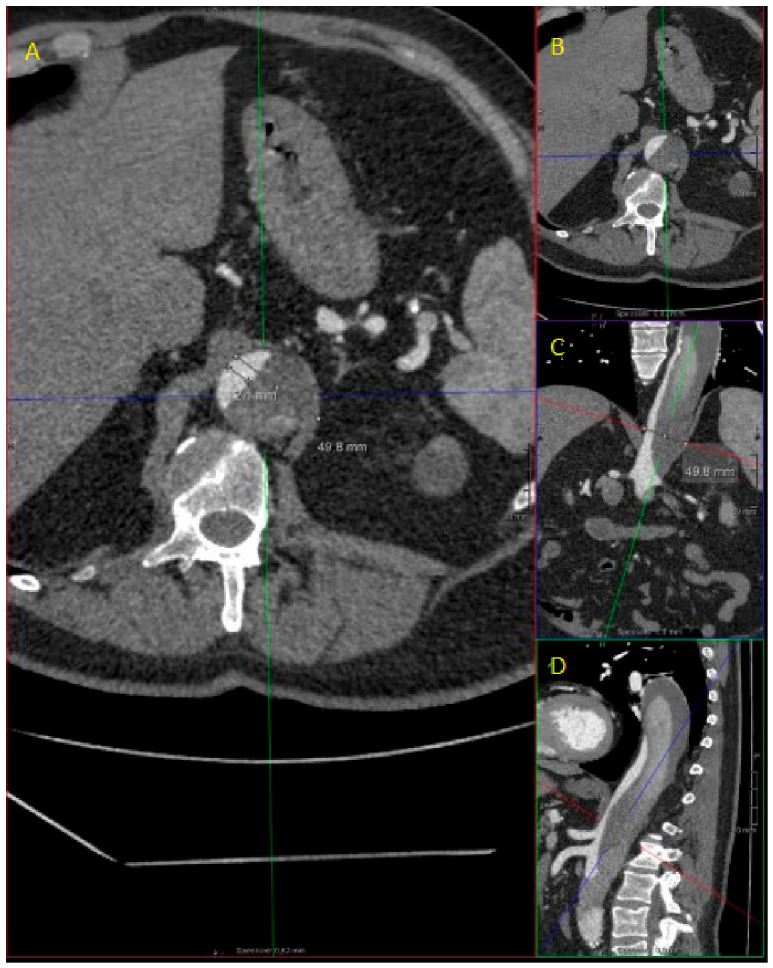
CT angiography ((A): axial; (B): axial at different level; (C): coronal and (D): sagittal) demonstrating chronic thoracoabdominal aortic dissection with persistent true and false lumen separation and associated mural hematoma. These findings reflect chronic wall remodeling and represent markers of ongoing wall fragility.
